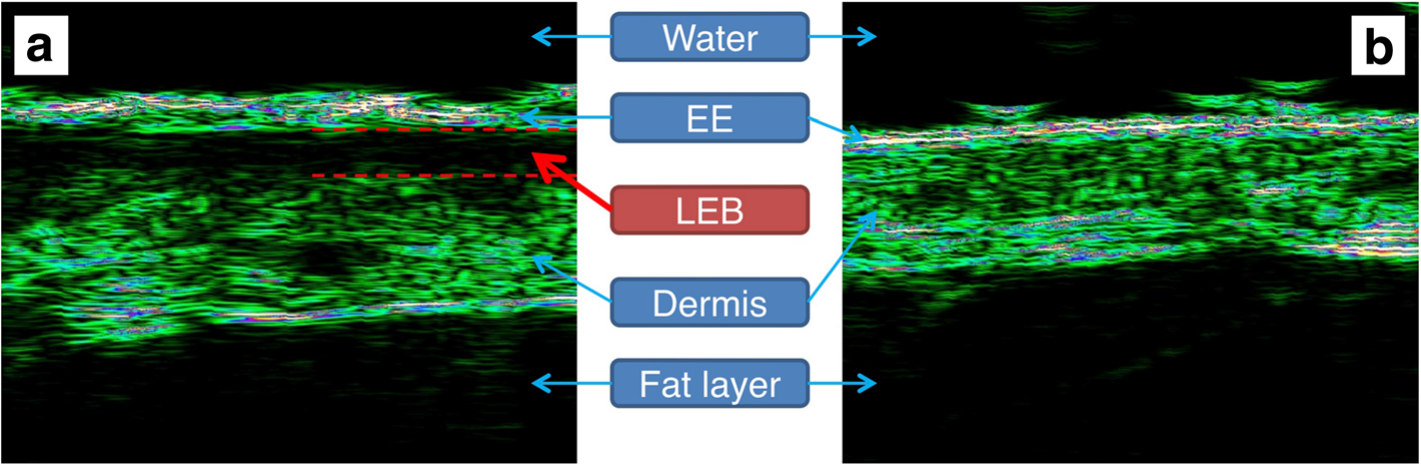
Ultrasonographic findings of the skin. a Re-epithelialized skin after a partial-thickness burn (thigh, healing time 19 days). b Contralateral normal skin. *EE* entrance echo, *LEB* low-echogenic band
